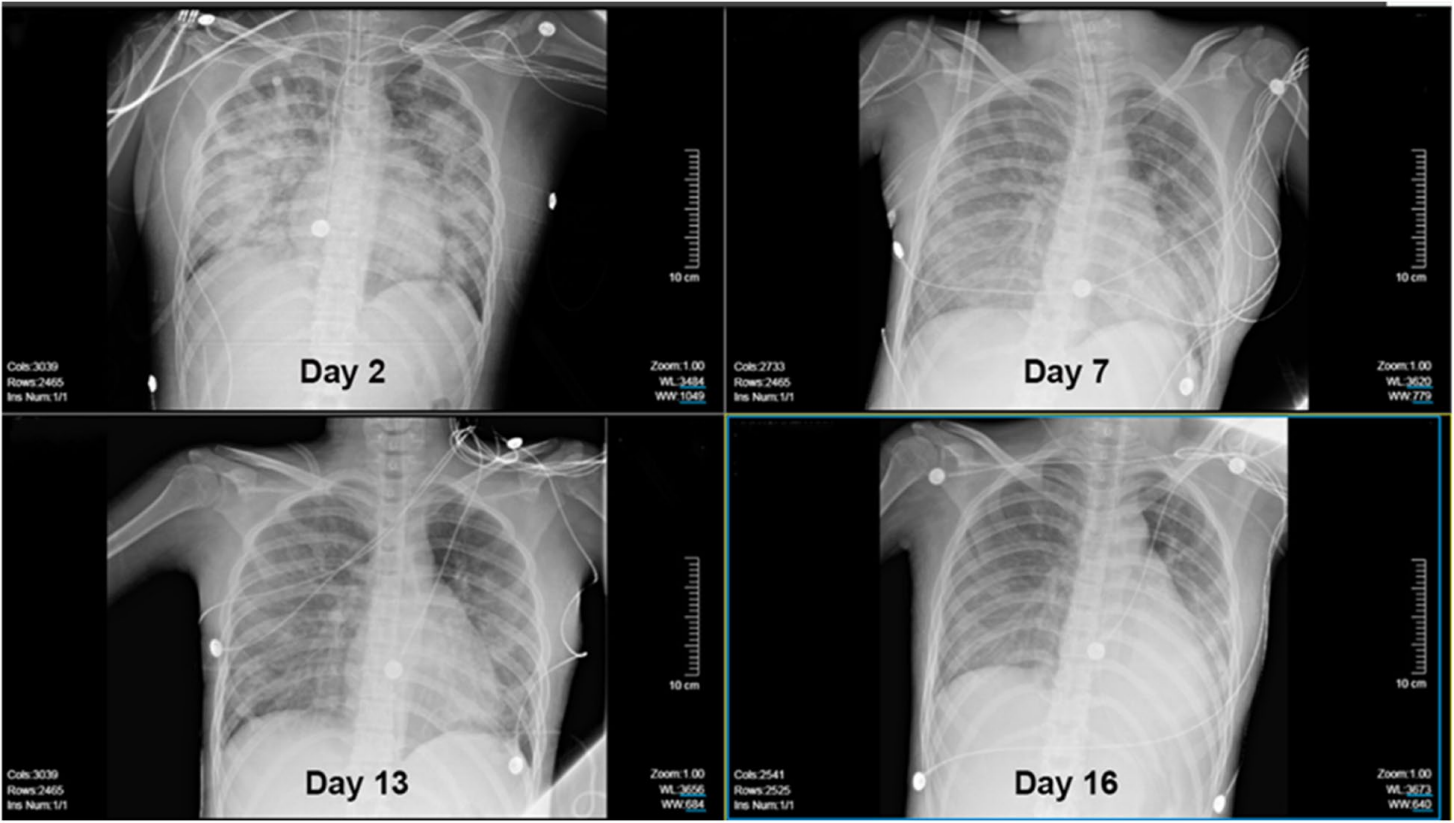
Chest X-ray during hospitalization
